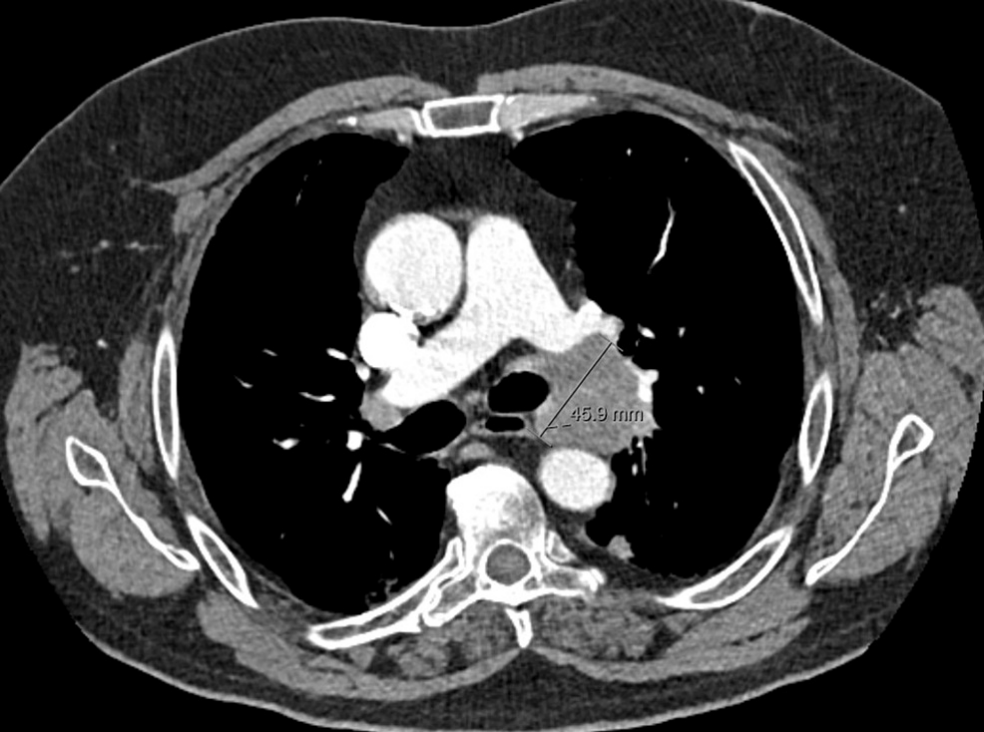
CT scan of the chest after first-line ChT, showing disease progression at the level of the left hilar lymph node conglomerate. CT: computed tomography; ChT: chemotherapy
